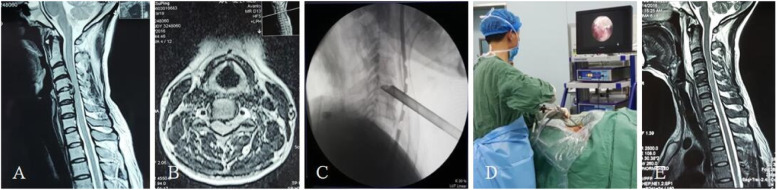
Percutaneous anterior full-endoscopic transcorporeal discectomy and spinal cord decompression. a The MRI sagittal image of the cervical spine showed C4/5 cervical disc herniation with local spinal stenosis and spinal cord compression. b The MRI axial image of the cervical spine showed C4/5 para-central cervical disc herniation. c Intraoperative radiography. d Intraoperative view. e Postoperative review of cervical spine MRI sagittal image showed complete removal of the C4/5 herniated disc tissue and complete decompression of spinal cord
